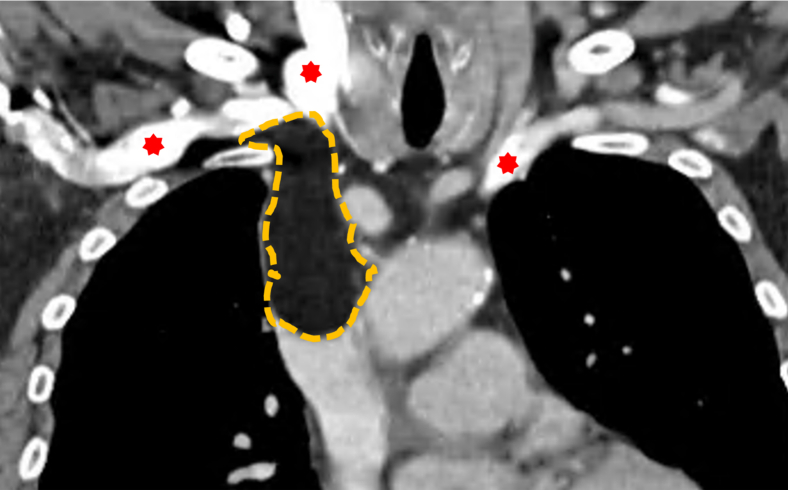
Preoperative computed tomography in venous phase. A voluminous hypodense mass fills the lumen of the superior vena cava in its cranial extent up to the brachiocephalic vein confluence. The *yellow dotted line* shows the edges of the mass. *Red points* mark the stasis of contrast agent in the supracaval veins.
